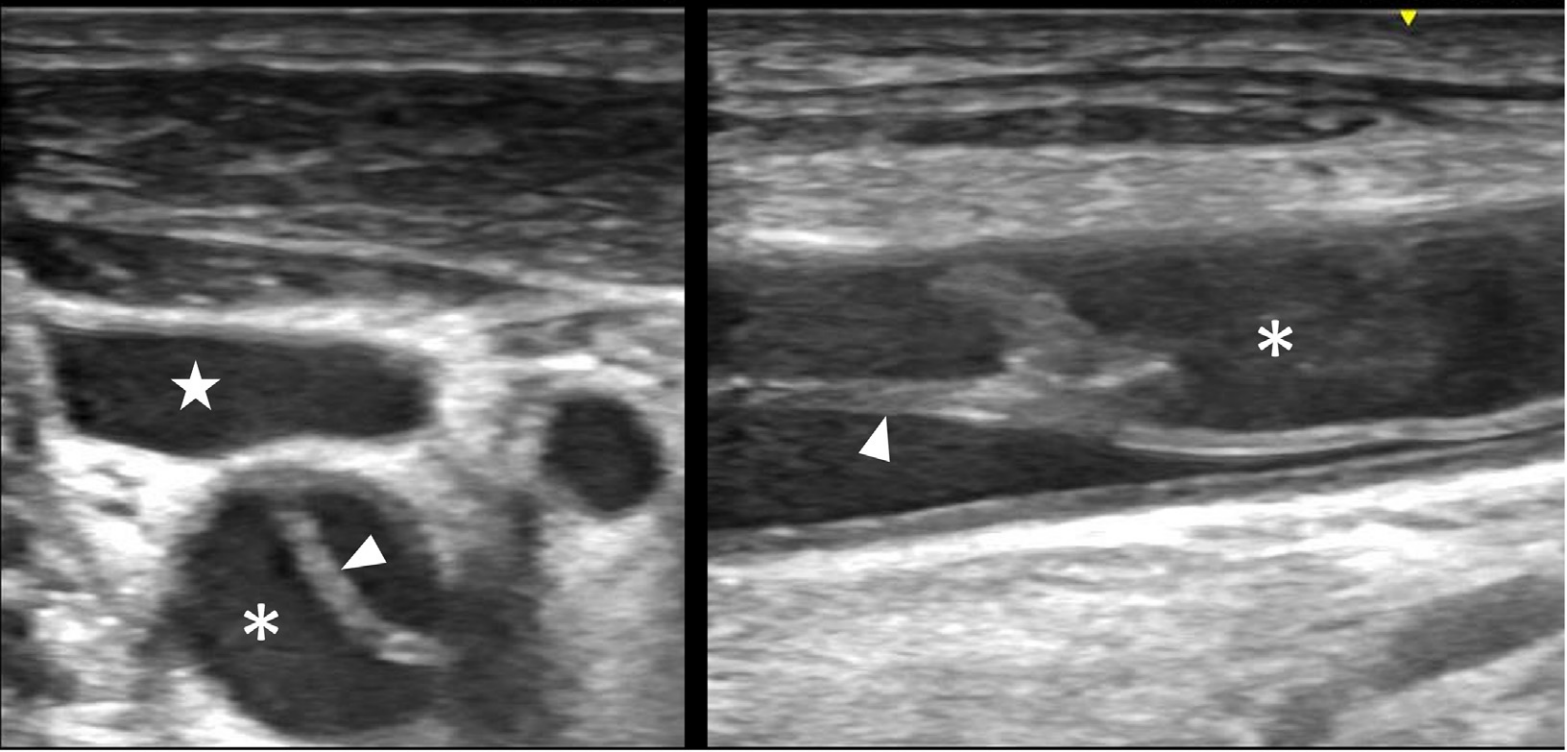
Transverse-axis (left panel) and long-axis (right panel) views of point-of-care right neck ultrasound demonstrating an internal jugular vein (star), carotid artery (asterisk), and echogenic linear carotid dissection flap (arrowhead).
